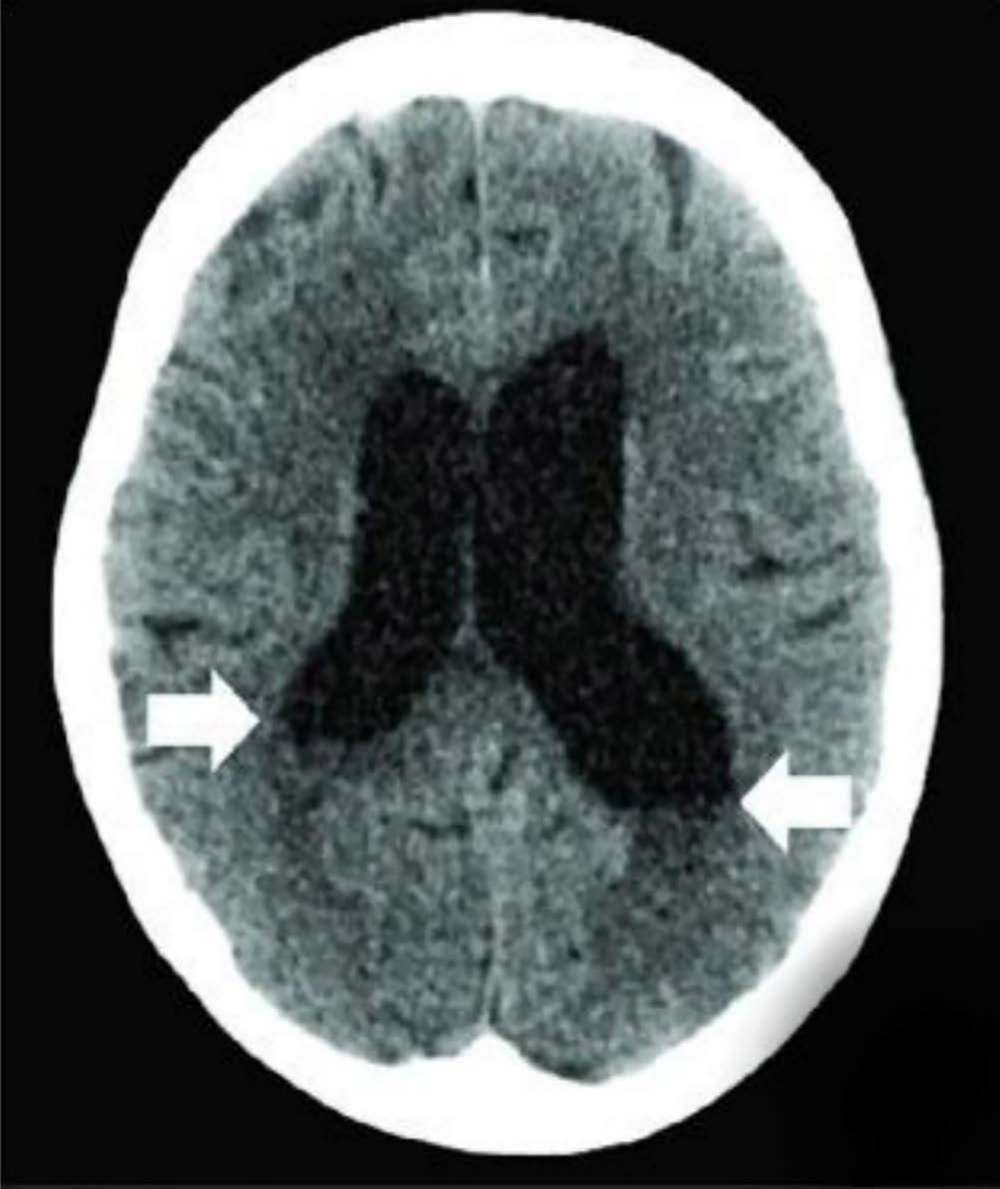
Axial CT image demonstrating ventricular dilation. White arrows indicate frontal horn width. The FOHR, calculated from this image, was estimated at approximately 0.5, consistent with moderate ventriculomegaly. CT = computerized tomography, FOHR = fronto-occipital horn ratio.
